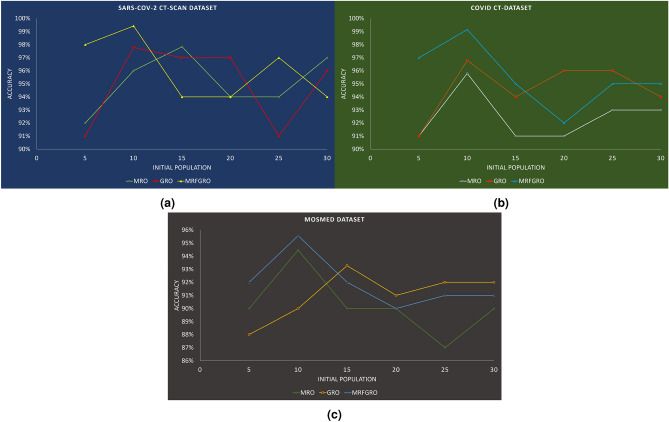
Graph showing the variation of classification accuracies with respect to various hyperparameters of proposed MRFGRO algorithm obtained on: (a) SARS-CoV-2 CT-Scan dataset, (b) Covid CT-dataset and (c) Mosmed dataset.
